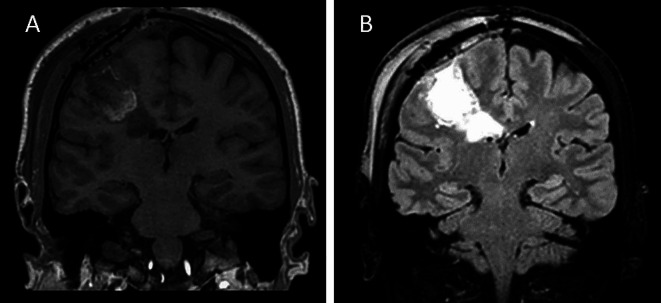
Brain magnetic resonance imagining showing a mass in the frontal lobe, inhomegenously intense in T1 weighted sequences (A) and hyperintense in T2 weighted sequences (B)
